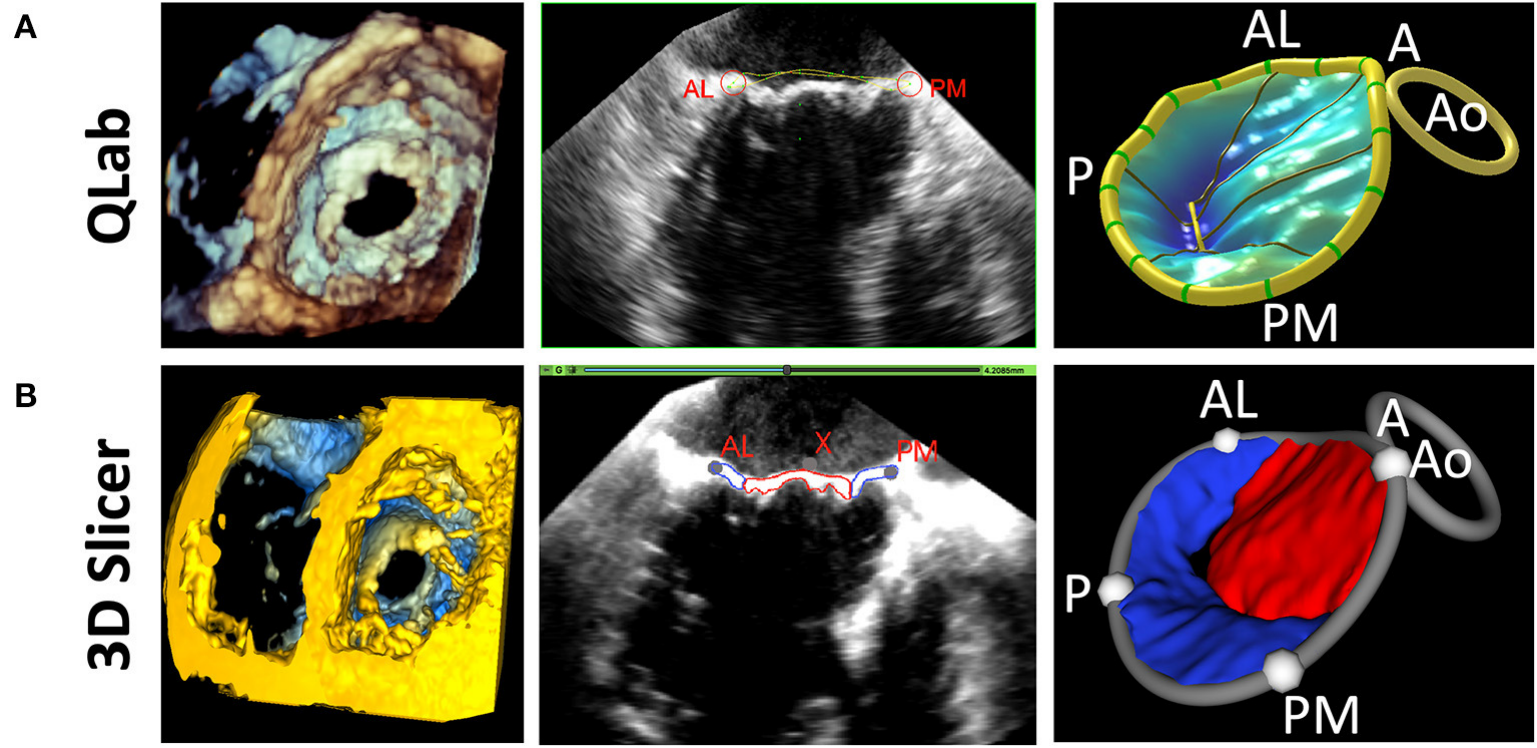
Comparison of 3D Slicer vs. QLab Mitral Valve Quantification (MVN). (Left) volume rendering of a mitral valve viewed from the left ventricle in Qlab (A) and 3D Slicer (B); 2D Transesophageal view of the mitral valve highlighting the AL and PM points in Qlab (A) and 3D Slicer (B); 3D Model generated in Qlab (A) and 3D Slicer (B). Qlab, Philips Medical, Andover, MA.
